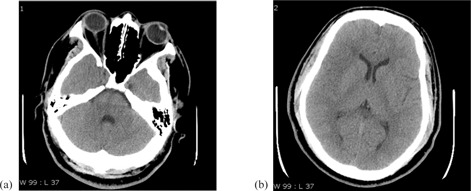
Representative head CT slices used in the study, at the level of (a) fourth ventricle, and (b) basal ganglia.
